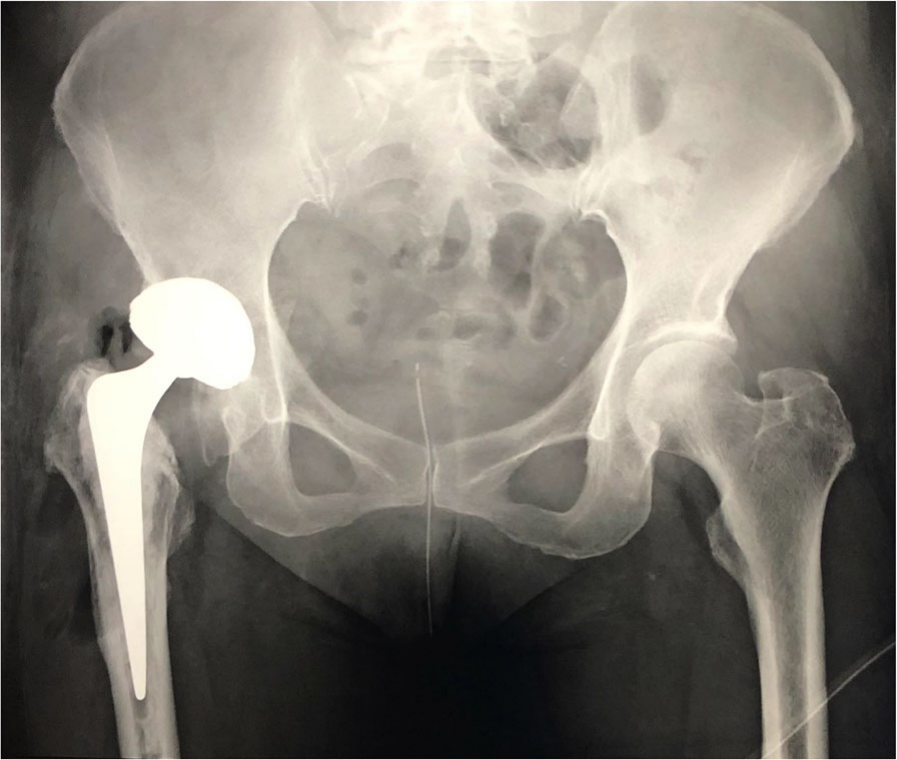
Postoperative radiography showing the cemented femoral stem
